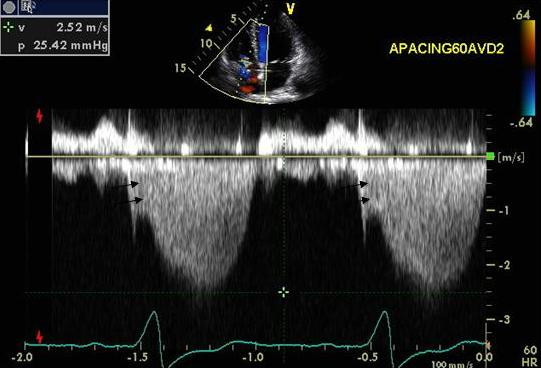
Continuous wave Doppler across tricuspid valve obtained during paced atrial rate of 60 bpm and atrioventricular delay of 220 ms showing significant diastolic tricuspid regurgitation (white arrow head).
